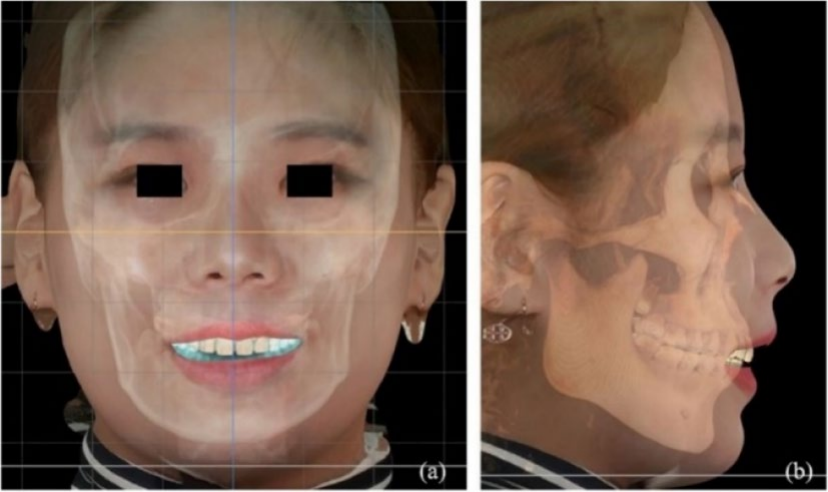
A digital twin reconstructed by the fusion of facial scan and CBCT images. a Coronal view of the face. b Sagittal view of the face [288]
